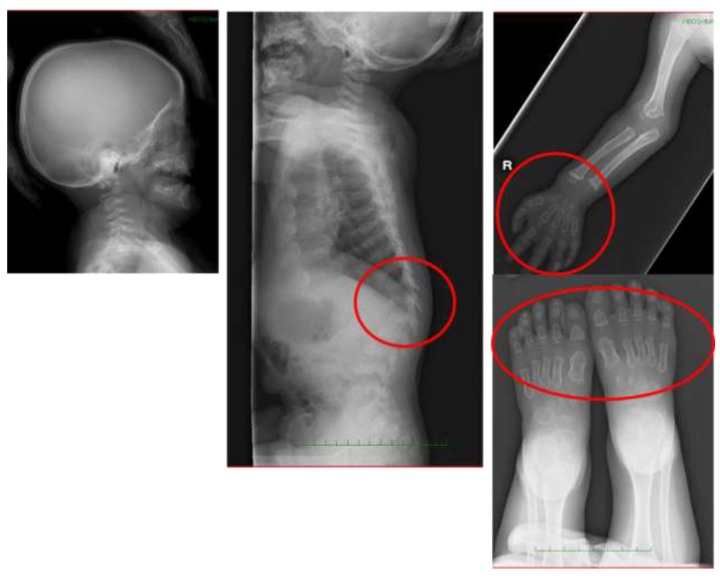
A whole-body X-ray from the first visit of a Japanese boy with MPS IVA. The findings of multiple dysostosis, such as the anterior tongue of the vertebrae and a dumbbell-like deformity in the proximal and middle phalanges of the hands and feet were observed.
